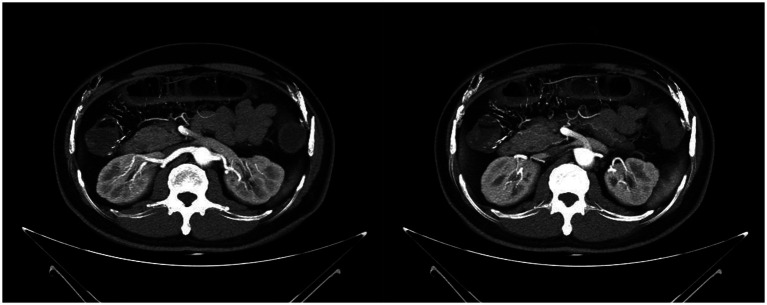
Computed tomography showed a thickened bowel wall with edema of the colonic wall in the right hemicolon, accompanied by multiple calcifications in the surrounding vasculature.
